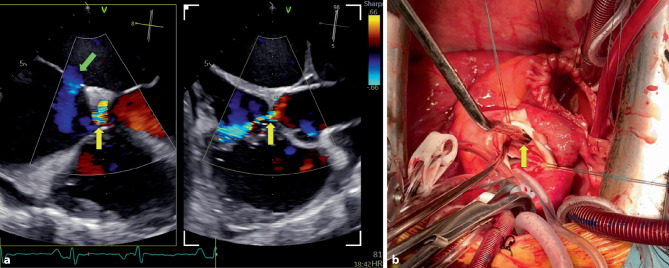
a Transesophageal echocardiography with color Doppler demonstrating an aorto-atrial fistula from the non-coronary sinus of Valsalva to the right atrium (*yellow arrows*) and a concomitant ostium secundum atrial septal defect (*green arrow*). b Intraoperative view showing the fistulous pathway (*yellow arrow*) during surgical repair
